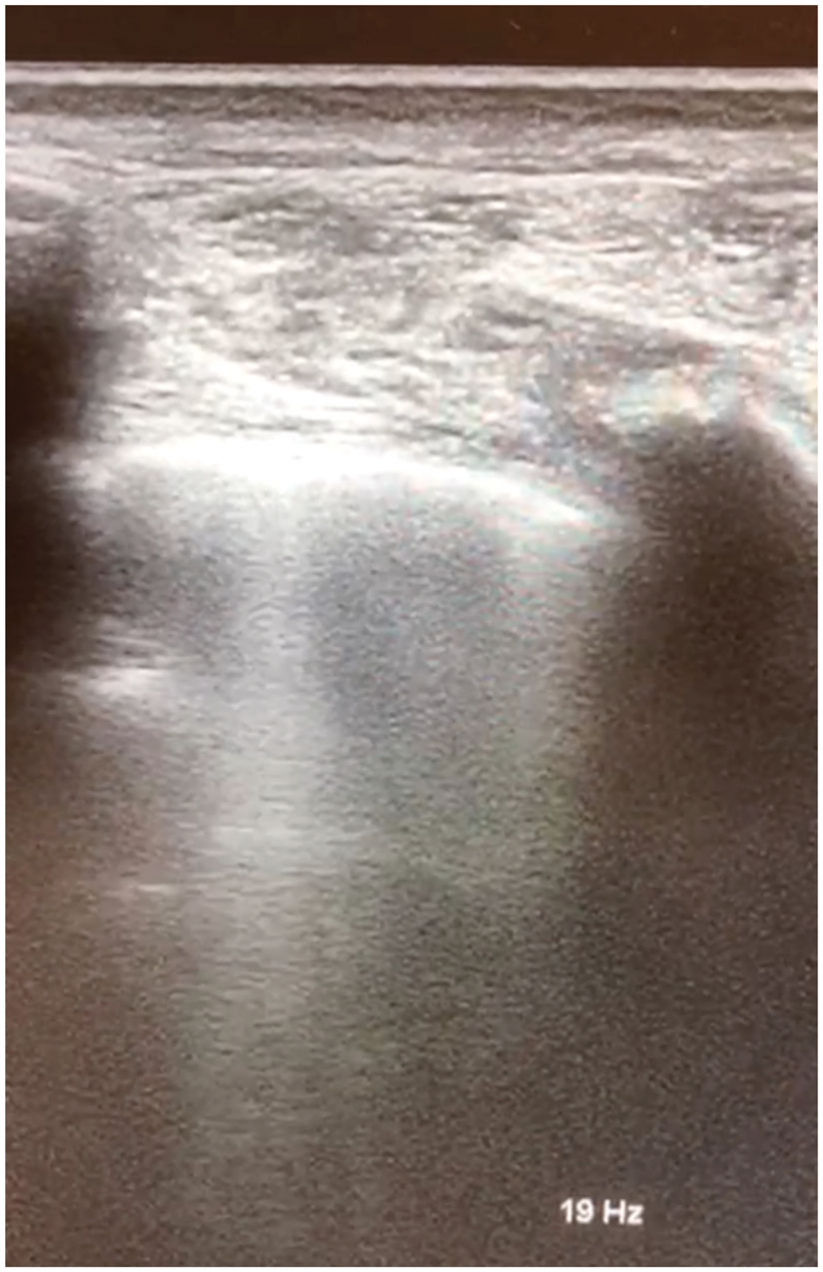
Bedside evaluation by a primary care physician of a patient with SARS-CoV-2 infection: B-lines at lung ultrasound appear as slightly hyperechoic bundles perpendicular to the hyperechoic pleural line.
